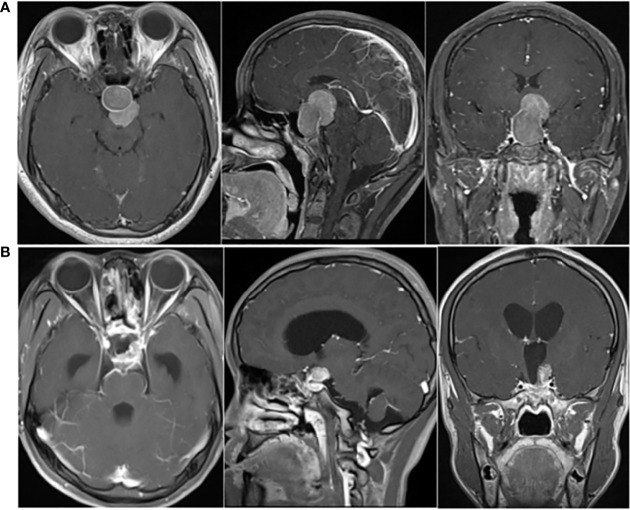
(A) Preoperative pituitary enhanced MRI (April 2016) showed: mass-shaped obvious enhanced signal could be seen in intrasellar and suprasellar area; the lesion extended upward to the suprasellar cistern, and the pituitary stalk was compressed, with the optic chiasm compressed and raised. (B) Postoperative pituitary enhanced MRI (October 2016) indicated: postoperative changes showed in the sellar region; abnormal enhancement of the upper left posterior pituitary appeared, with left cavernous sinus closely related.
